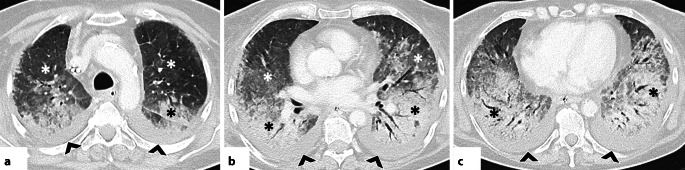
a–c Axiale Aufnahmen der hochauflösenden Computertomographie (HRCT): diffuse Milchglasverdichtungen (*weiße Sternchen*) in Kombination mit ausgedehnten Konsolidierungen (*schwarze Sternchen*), vorwiegend in den Unterlappen und abhängigen Lungenabschnitten. Zudem ist beidseitig ein Pleuraerguss erkennbar (*schwarze Pfeilköpfe*). Die Befunde entsprechen einem diffusen alveolären Schaden (DAD) im Rahmen einer vorbestehenden systemischen Sklerose
